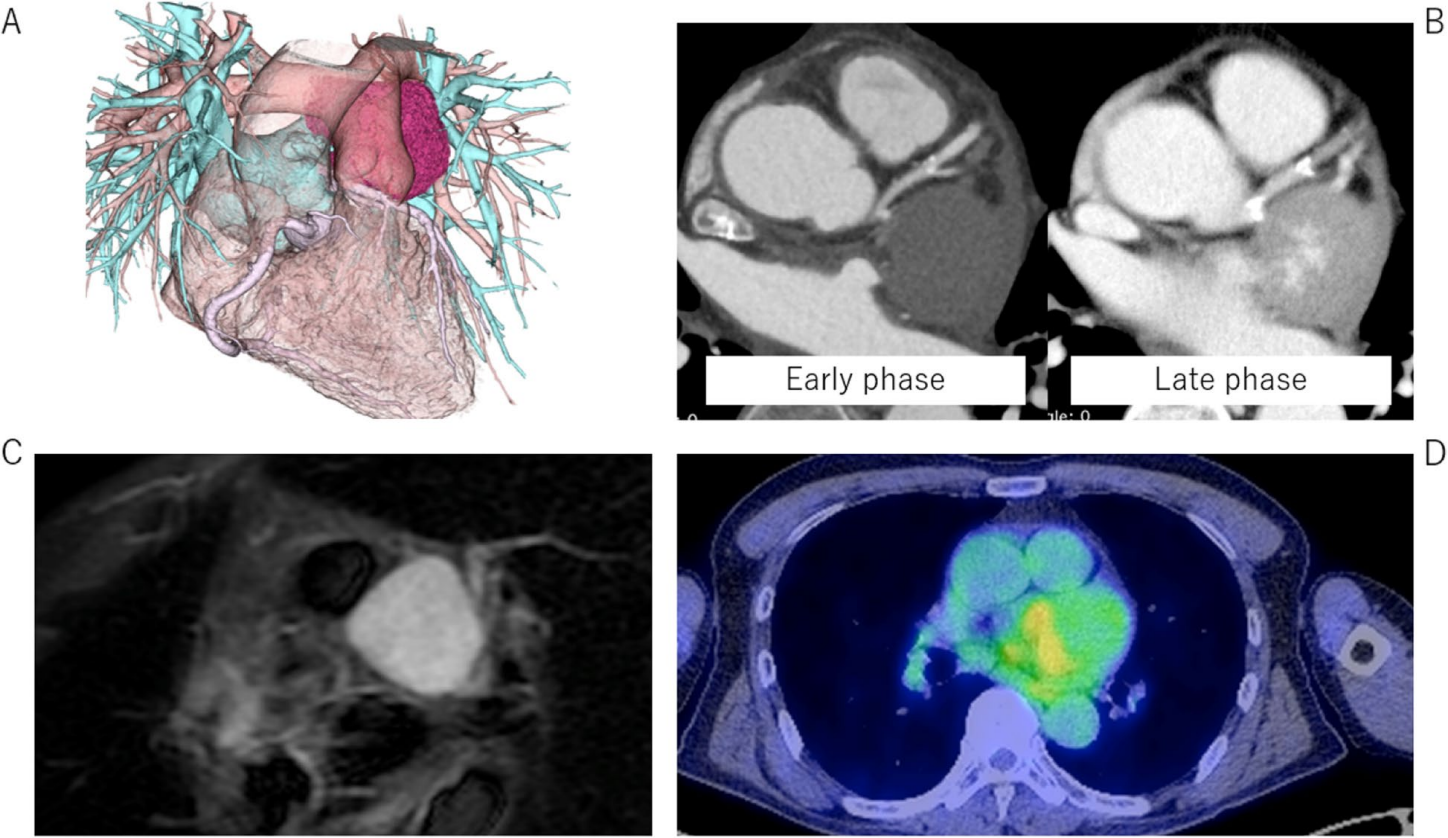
A Preoperative computed tomography (CT) imaging showed a tumor with a size of approximately 60 mm. B The tumor was located between the left atrium and epicardium. Contrast medium spread more widely in the tumor in the late phase than in the early phase. C The tumor showed high signal intensity on magnetic resonance imaging (MRI) T2-weighted image. D The tumor demonstrated high uptake of F-18-deoxyglucose (FDG) on positron emission tomography (PET)/CT (white arrow)
